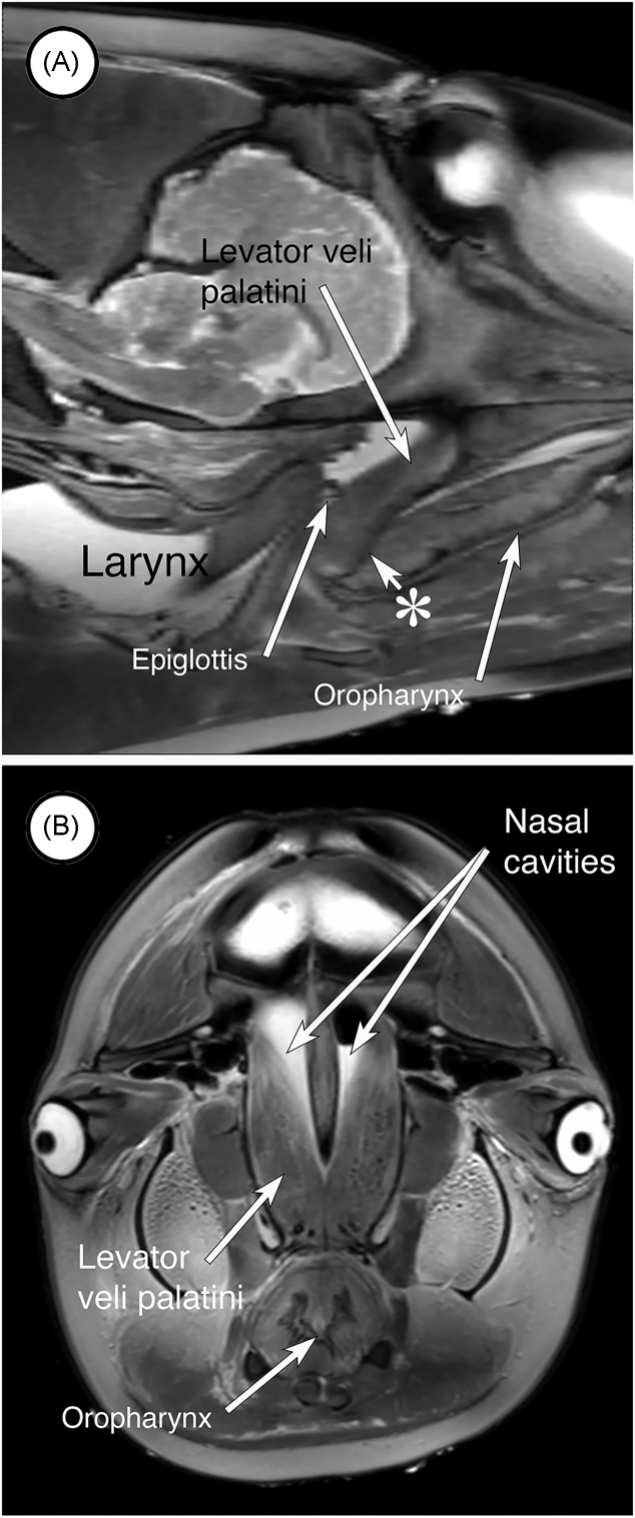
MRI scans in the sagittal (A) and transverse (B) planes showing the levator veli palatini muscles. (A) Shown here is the levator veli palatini muscle emerging from one of the nasal cavities and merging with the rostral aspect of the palatopharyngeal sphincter. A layer of fascia separates (arrow labelled with the asterisk) components of parts of the sphincter rostral to the epiglottis of the larynx and may represent remnants of the aponeurosis of tensor veli palatini. (B) The levator veli palatini muscle on each originates high on the lateral wall of the nasal cavity.
